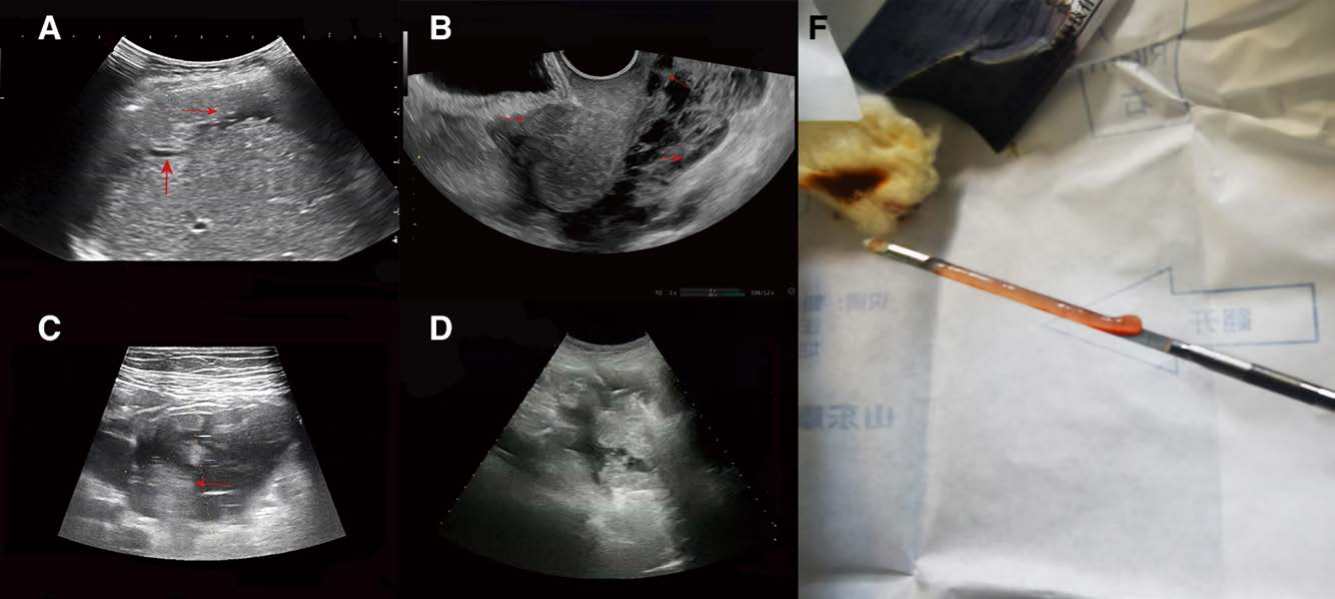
(A) Thickening of the perihepatic peritoneum, with finger-pressure signs visible on the surface of the liver. (B)Nodules on the plasma surface of the uterus, foveal fluid dark areas in the cysto-uterine fossa and the rectouterine pouch, and diffuse moderate echogenicity. (C) Hypoechoic mass in the ileocecal region with visible nodules. (D) Ultrasound-guided puncture. (E) Tissue strip.
